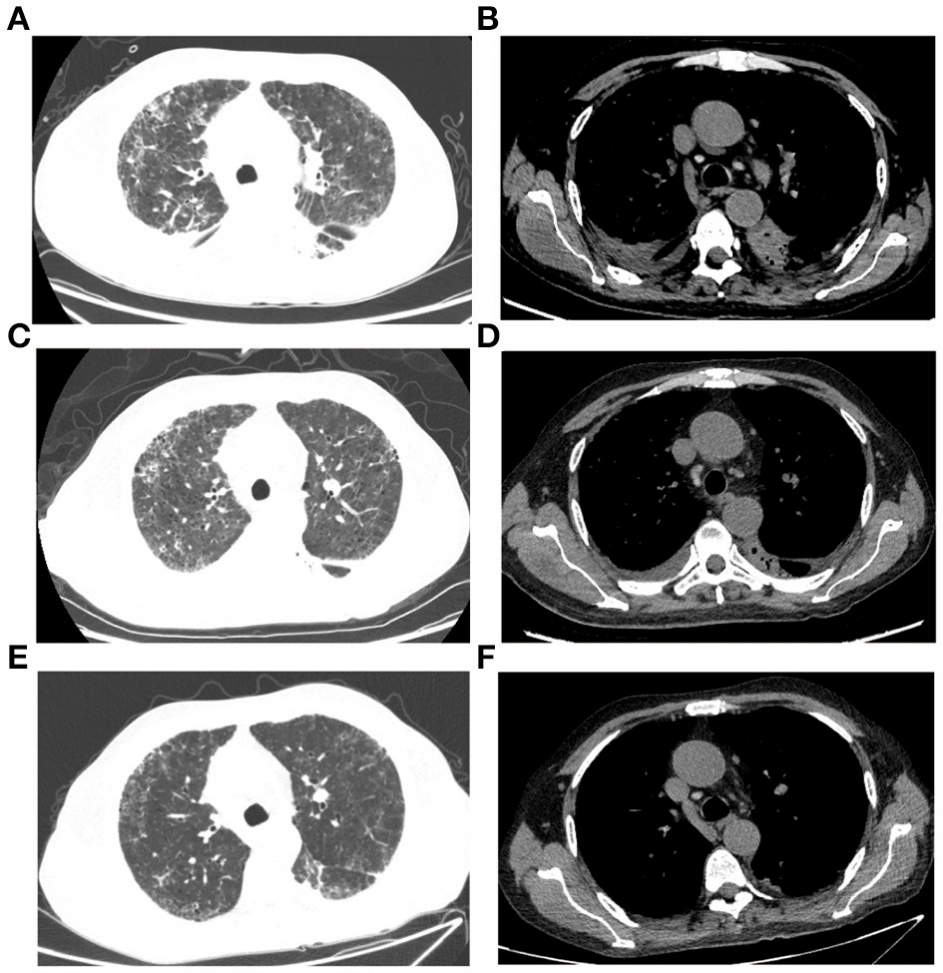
Chest CT imaging of the patient. (A,B) CT imaging on October 22, 2020; (C,D) CT imaging on November 3, 2020; (E,F) CT imaging on December 6, 2020.
